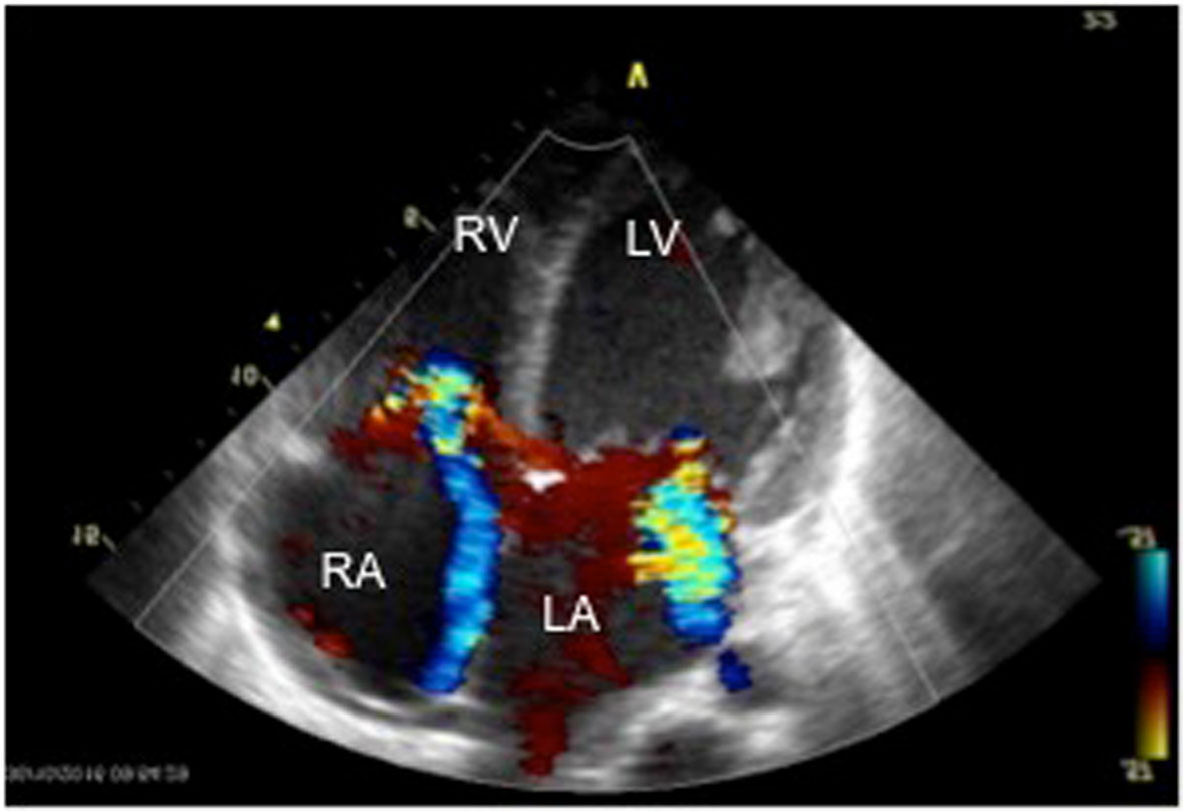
A four-chamber dilative cardiomyopathy with biventricular dysfunction (left ventricular ejection fraction 18%) was diagnosed 13 weeks after the accident. The echocardiography findings showed: enlargement of all the heart cavities; a left ventricular end diastolic diameter 65 mm (normal values 46.8 ± 6 mm); ejection fraction 18% (normal values 50–65%); tricuspid annular plane systolic excursion 1 cm (normal values > 1.6 cm); distinct central mitral insufficiency; and moderate tricuspid insufficiency. There was also a high suspicion of secondary pulmonary hypertension in the context of left ventricular insufficiency (systolic pulmonary artery pressure 45 mm Hg + central venous pressure). *LA* left atrium, *LV* left ventricle, *RA* right atrium, *RV* right ventricle
